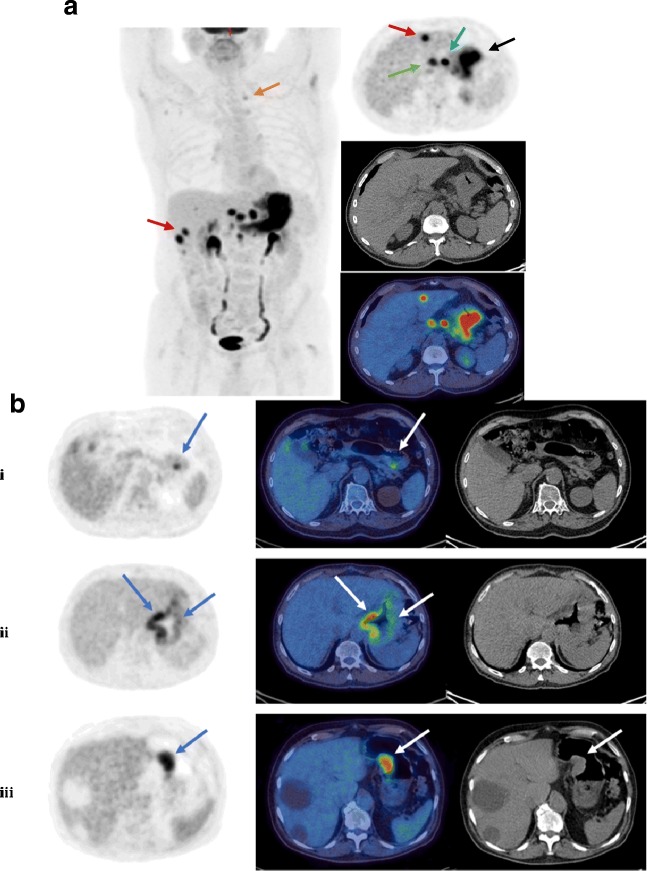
Example FDG PET-CT images. a*Left*: maximum intensity projection (MIP) showing diffuse gastric malignancy with multiple left gastric, coeliac, portal and retroperitoneal nodes, bilobar liver metastases and a metastatic superior mediastinal node. *Right*: axial (PET – *top*, CT – *middle*, fused PET-CT – *bottom*). *Arrows* indicate the gastric primary (*black*), a regional left gastric node (*blue*), bilobar liver metastases (*red*), a portal node (*green*) and a small left superior mediastinal node (*orange; left image*). b Example FDG PET-CT images from three patients with different uptake distribution. Shown are axial images, from left to right: MIP, fused PET-CT, CT only; (i) Focal primary tumour uptake, lesser curvature of stomach, (ii) Diffuse primary tumour uptake along both greater and lesser curvatures of stomach, (iii) Lesion along lesser curvature of stomach corresponding to soft tissue on CT. Note photopaenic large simple hepatic cysts
